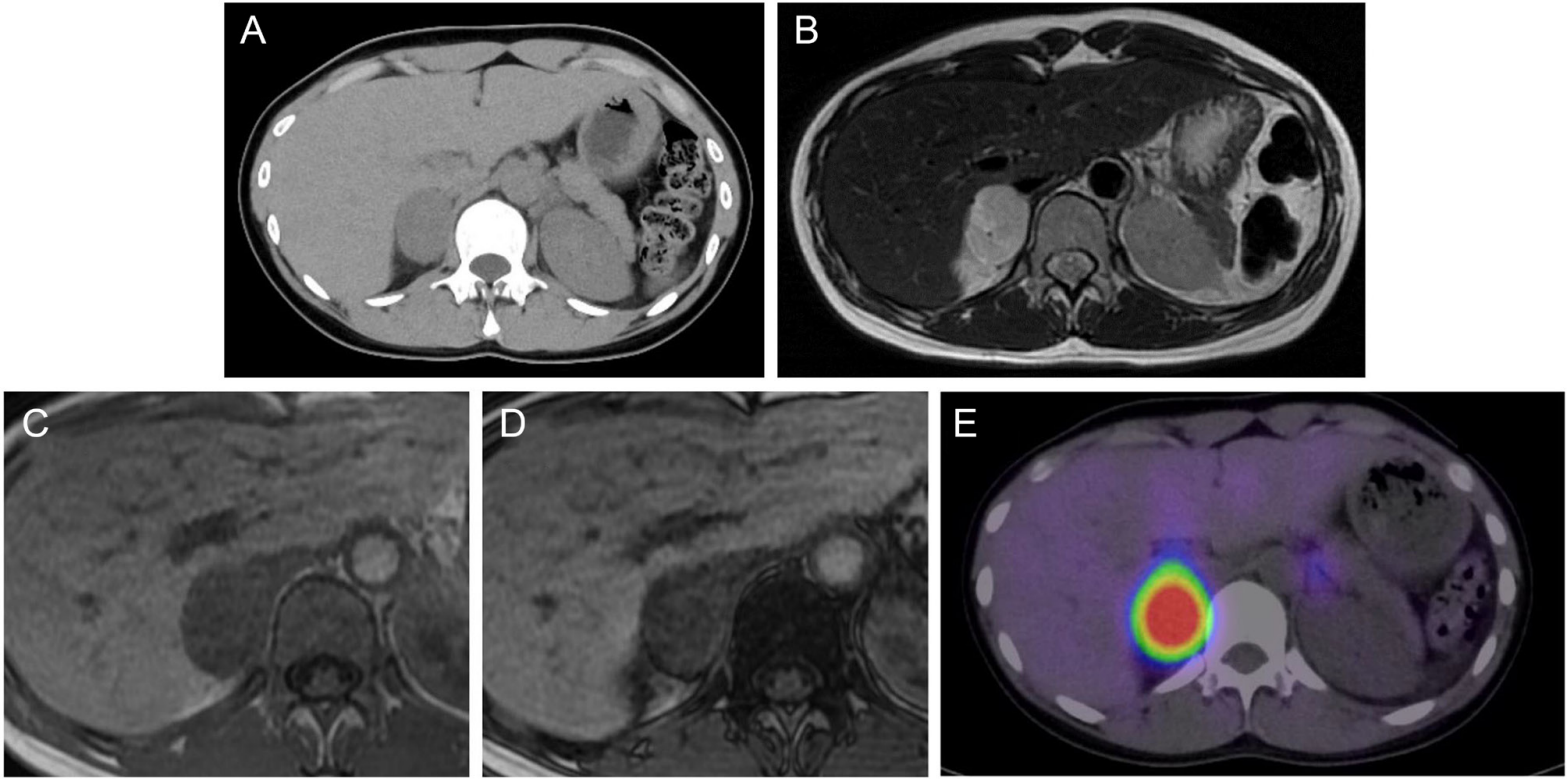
Images of the right adrenal tumor. (A) Right adrenal tumor measuring 38 × 26 mm (computed tomography number: 40 HU). (B–D) Right adrenal tumor showing high signal on T2-weighted images and no signal loss on T1-weighted images from the in-phase to the out-of-phase. (B) T2-weighted images. (C) T1-weighted images (in-phase). (D) T1-weighted images (out-of-phase). (E) Iodine-123 metaiodobenzylguanidine scintigraphy showing high uptake, consistent with the finding of a tumor.
